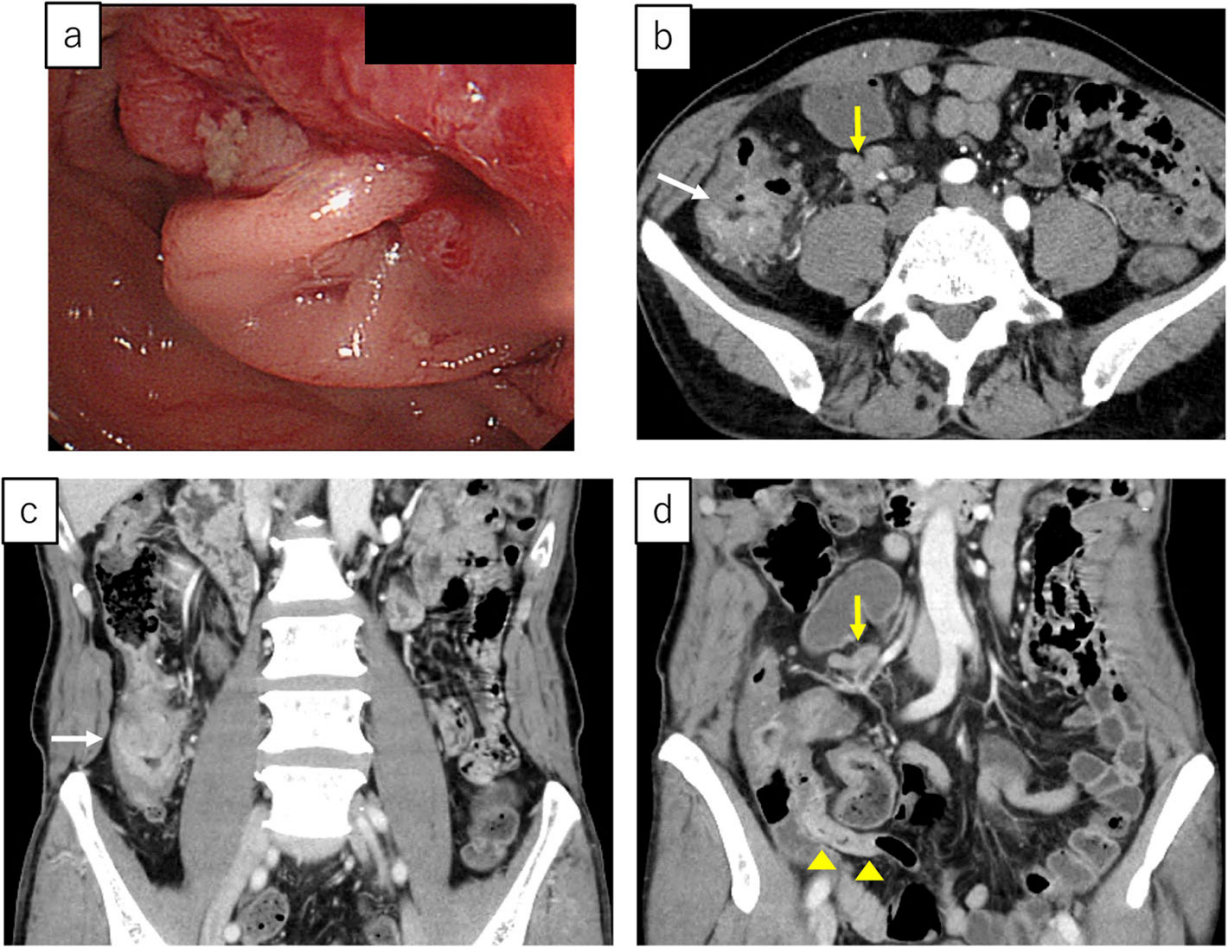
a Colonoscopy image showing a type-2 tumor in the ascending colon. b–d Enhanced abdominal computed tomography images showing focal thickening in the ascending colon (white arrow) and middle of the appendix (yellow arrowheads) and swelling of the lymph nodes around the ileocolic artery (yellow arrow)
